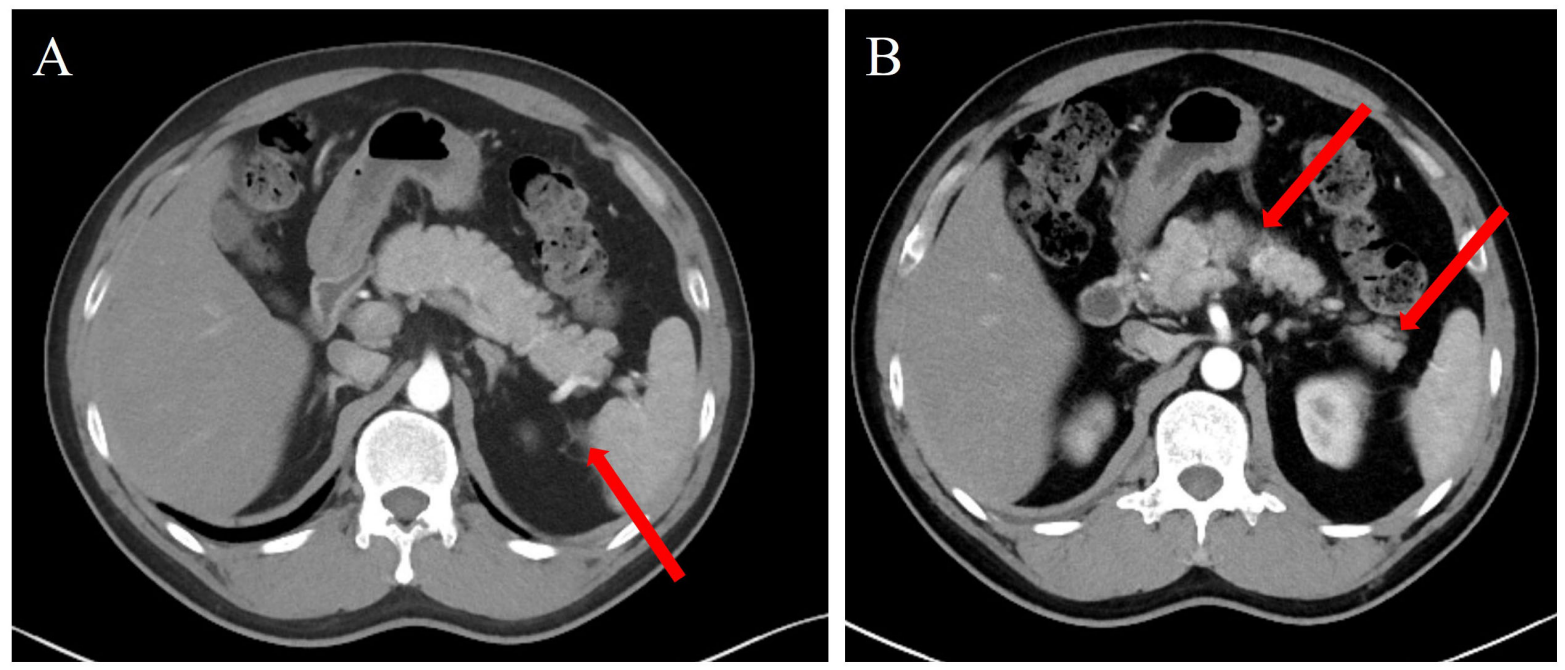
(A, B) Computed tomography images show pancreatic edema and surrounding inflammatory exudation. The area indicated by the red arrow demonstrates peripancreatic inflammatory exudate; however, the overall morphology of the pancreas remains intact, indicating mild pancreatitis-related injury.
